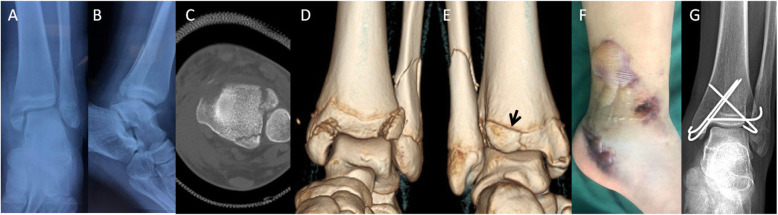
(Case 6): a AP radiograph showed distal fibular fracture and MMF associated. b Lateral view showed a radiolucent zone consistent with a fracture at the posterior aspect of the tibia, but it was not clear because of superimposition of the fibula. c, d, and e Axial CT section and 3D showed Tillaux fragment and APITEL (black arrow). f General observation showed significant edema of the soft tissue. g AP plain radiograph obtained 7 months after treatment showed complete reduction of medial joint space immediately post operatively
